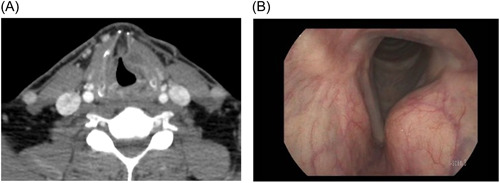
Preoperative views of neoplasm. (A) Axial section of computed tomography scans at the level of the thyroid cartilage, demonstrating well‐circumscribed homogeneous hypodense lesion within the left thyroid ala. (B) Flexible laryngoscopy demonstrating supraglottic submucosal mass.
